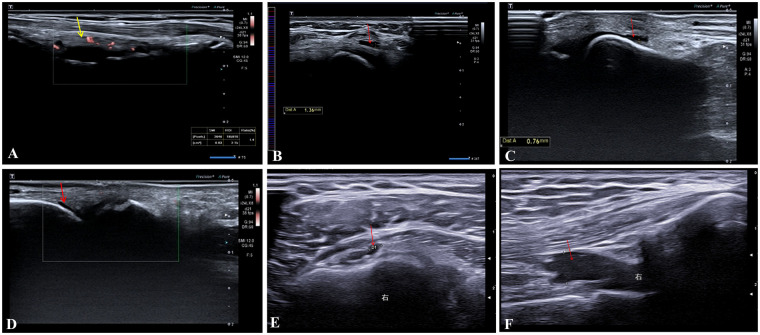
Musculoskeletal ultrasonography findings. (A) Synovitis of the wrist joint; (B) Effusion in the wrist joint; (C) Effusion in the metacarpophalangeal joints; (D) Effusion in the proximal interphalangeal joints; (E) Effusion in the shoulder joint; (F) Effusion in the suprapatellar Bursa of the knee. Overall, grade 1 synovitis with effusion was identified in multiple peripheral joints.
